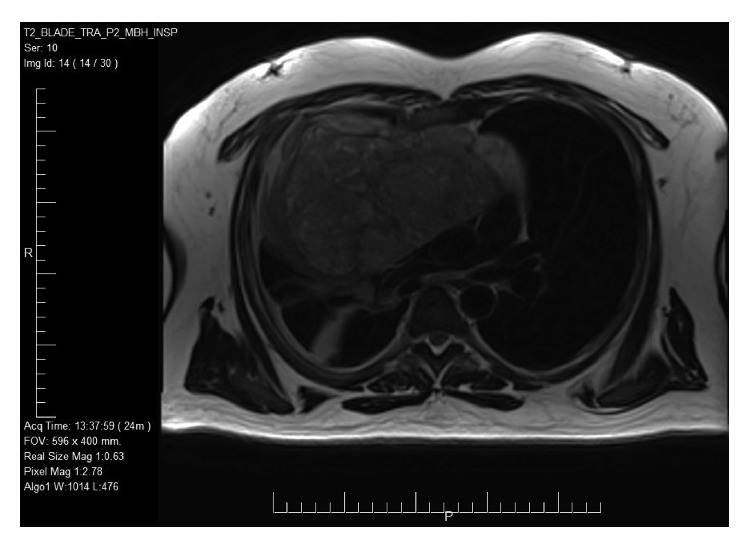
A large mediastinal mass is noted on MRI before surgery.
